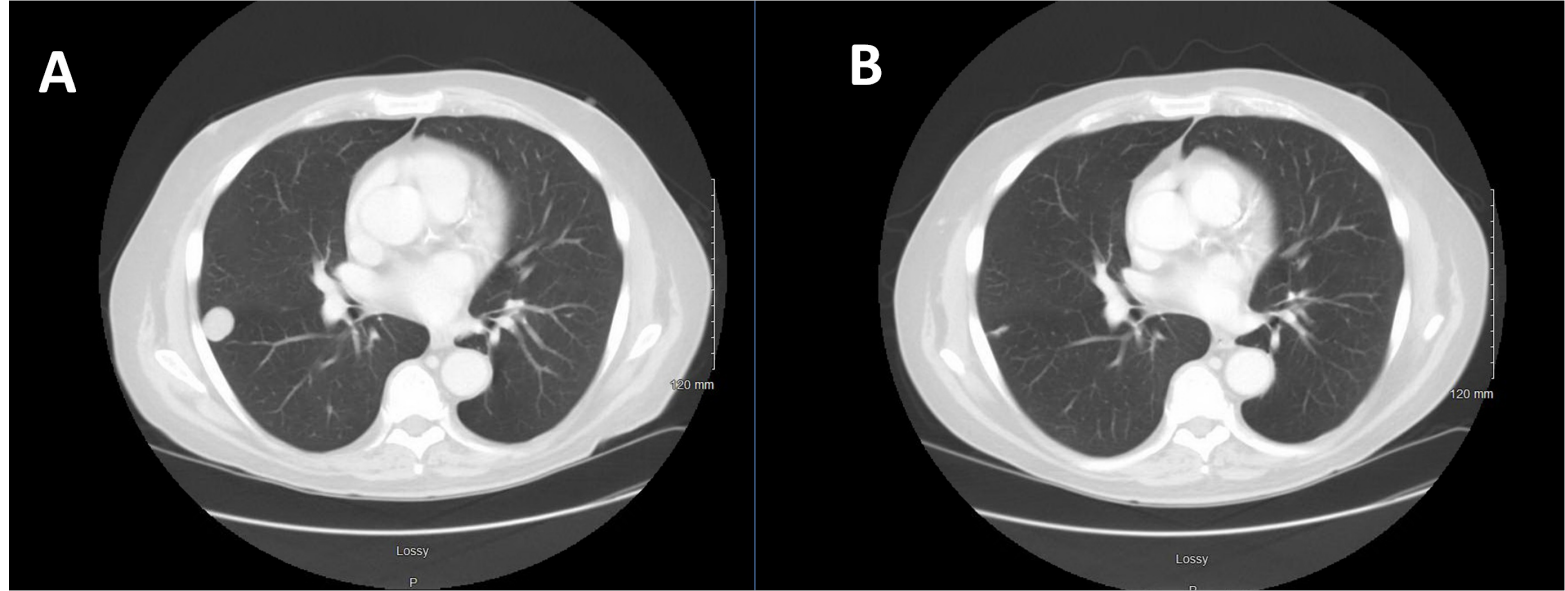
CT Chest Before and After Six Weeks of BRAF-targeted Therapy A: CT scan of the chest after receiving a total of three cycles of combination chemotherapy (gemcitabine/docetaxel) and prior to BRAF-targeted therapy. B: CT scan of the chest after six weeks of dabrafenib/trametinib therapy, revealing regression of the right pulmonary metastasis.
